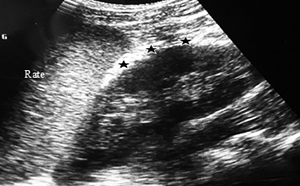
Ultrasound image of a small subcapsular hematoma of the left kidney. Hyperechoic arcuate border (black stars) between the spleen (*rate* in French) and kidney.
